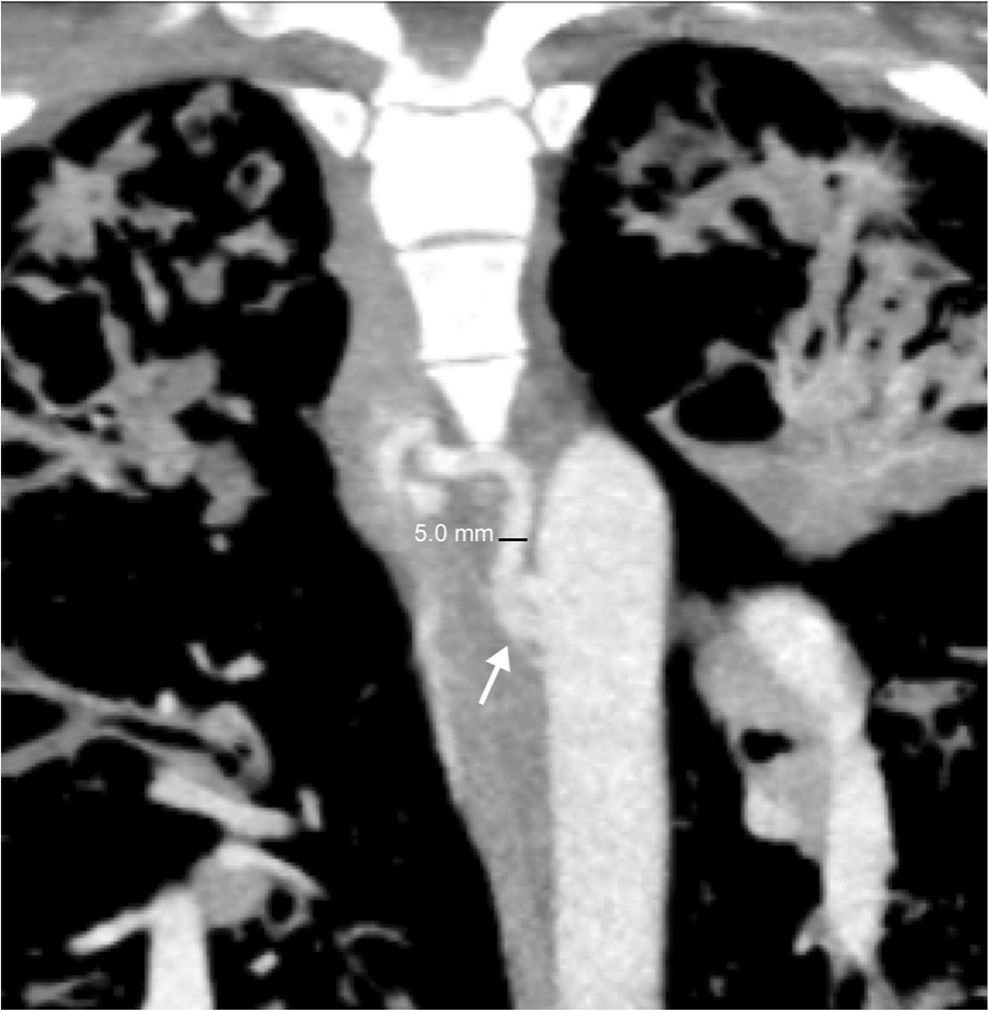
Bronchial artery anatomy. Coronal CT of the chest shows a dilated right bronchial artery (5 mm) arising from the intercostal bronchial artery trunk (ICBAT) (*white arrow*) of the postero-lateral thoracic aorta at the level of T6. Please note that the normal bronchial artery is less than 2 mm in diameter. While ICBAT origin is the most typical of the right bronchial artery, the left bronchial arteries usually arise directly from the aorta
